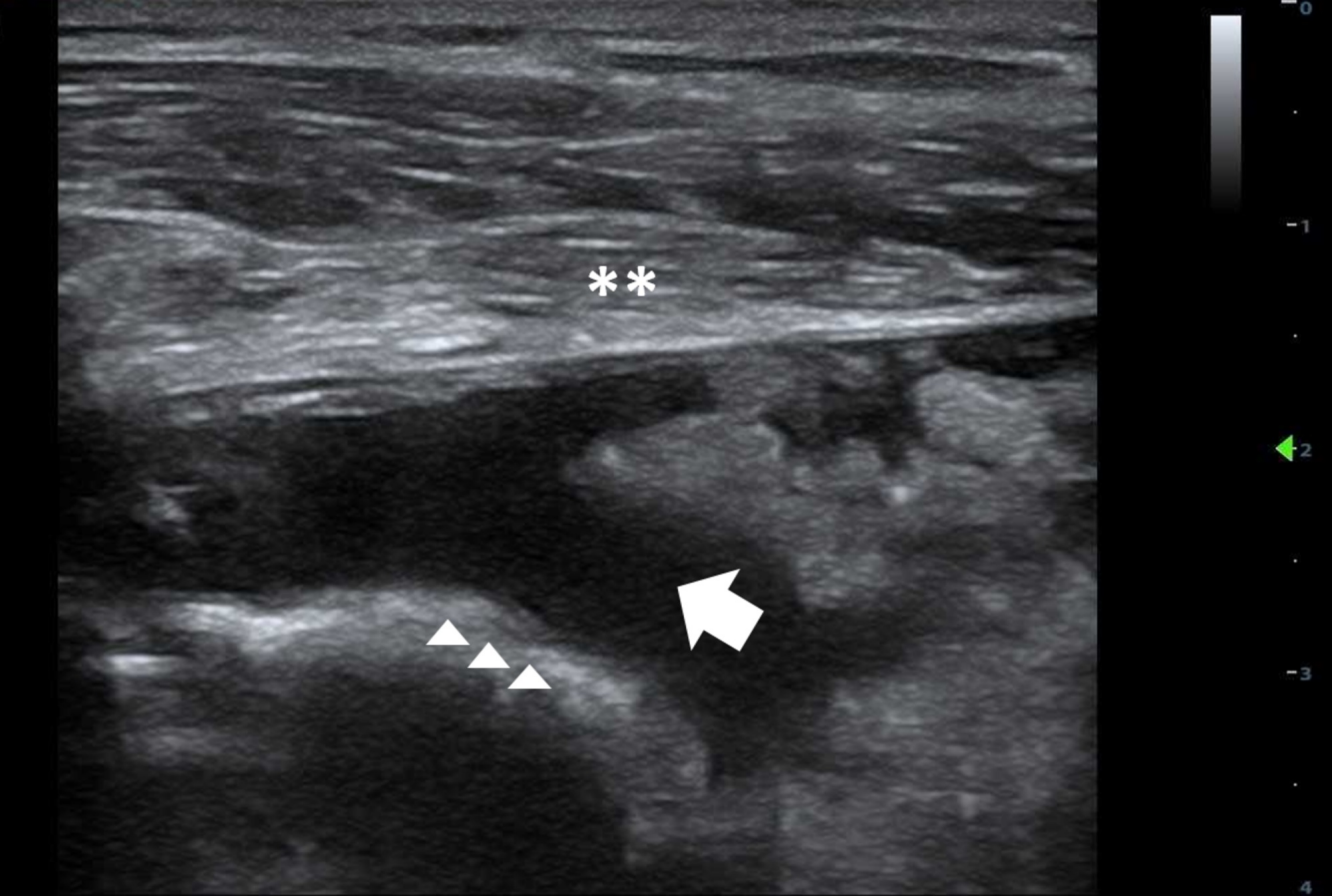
Right shoulder ultrasound showing fluid collection - deltoid ^^^- humeral head
